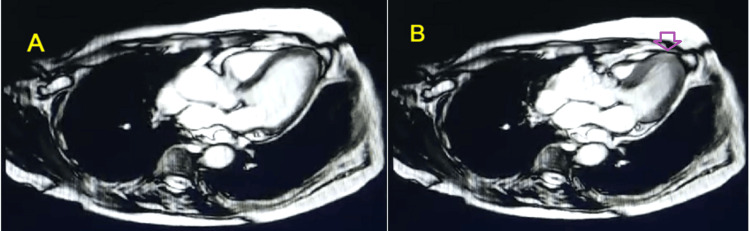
Axial MRI images in diastole (A) and systole (B) showing complete recovery of left ventricular function, with the arrow pointing to a normokinetic left ventricular apex
